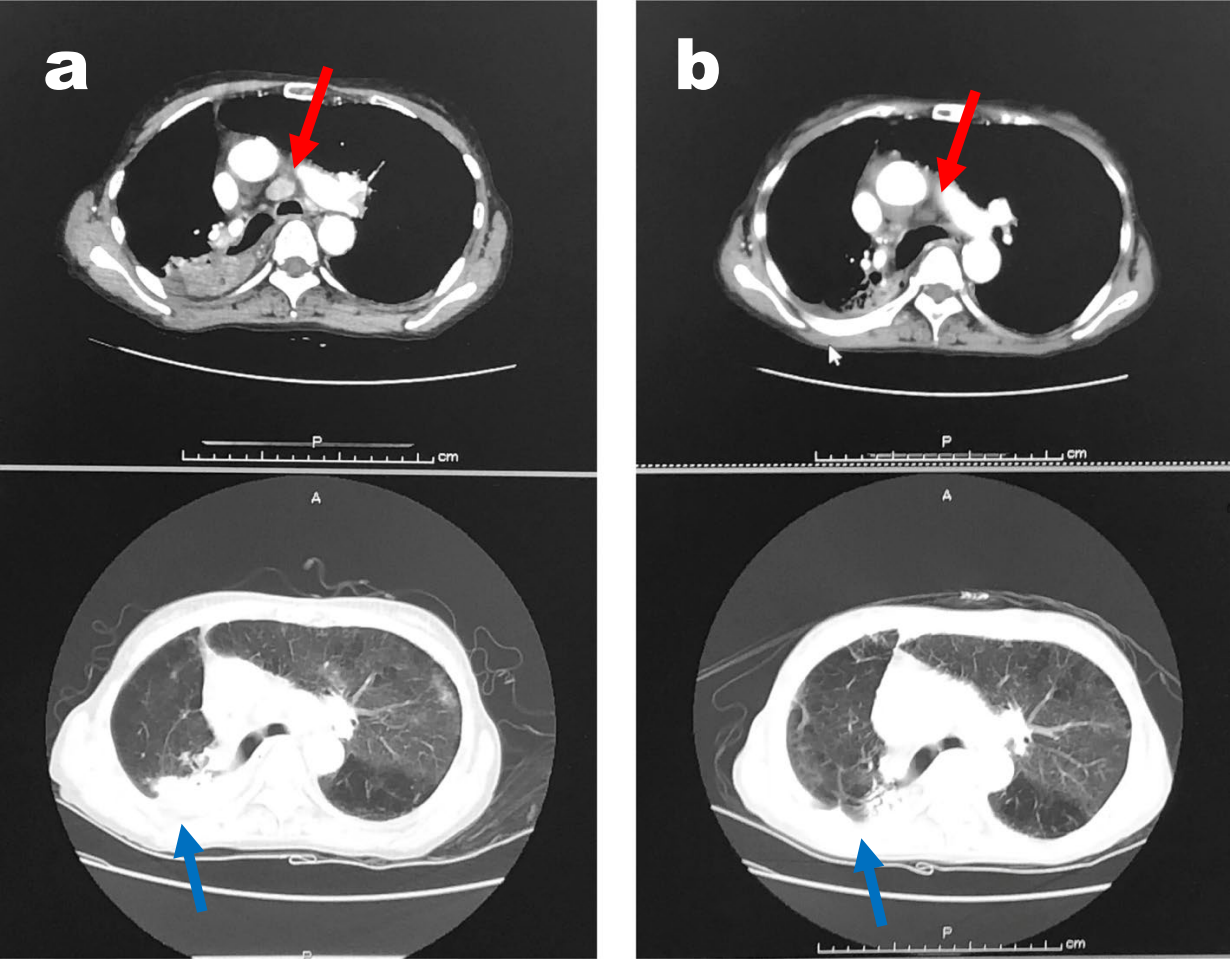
Chest CT scan Chest computed tomography with iodine-based contrast medium. Axial scans: (a) prior to, and (b) after immuno-chemotherapy. Both involved lymph nodes (red arrows) and the primary lung lesion (blue arrows) are smaller after the immuno-chemotherapy
